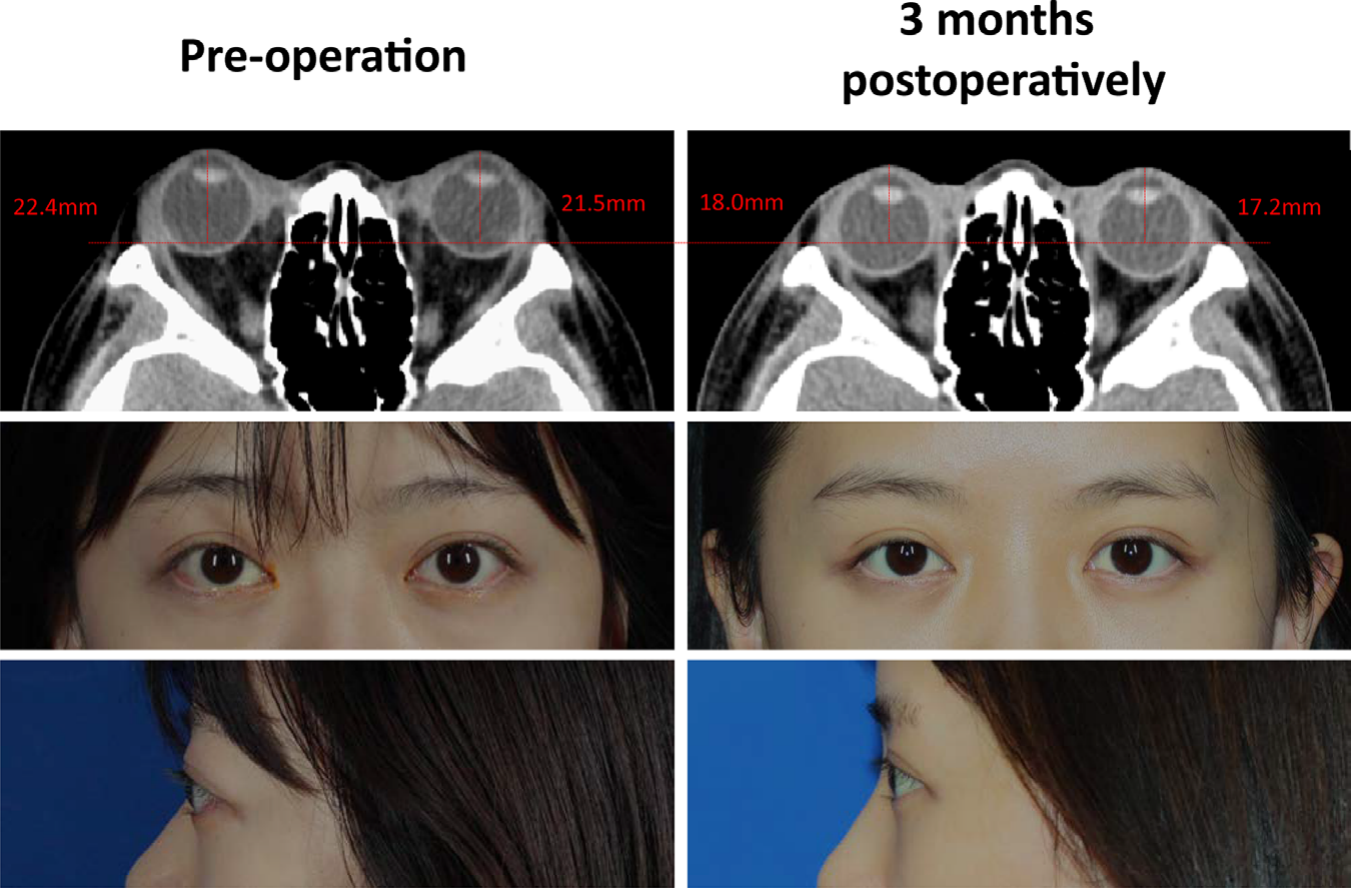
An overview of one patient with good curative effect. The orbital CT showed that the orbital fat tissue was reduced and both eyes were retracted into the orbit. The proptosis and eyelid retraction symptoms were both relieved after the surgery. A statement of consent to publish the results and images was gathered from the patient.
